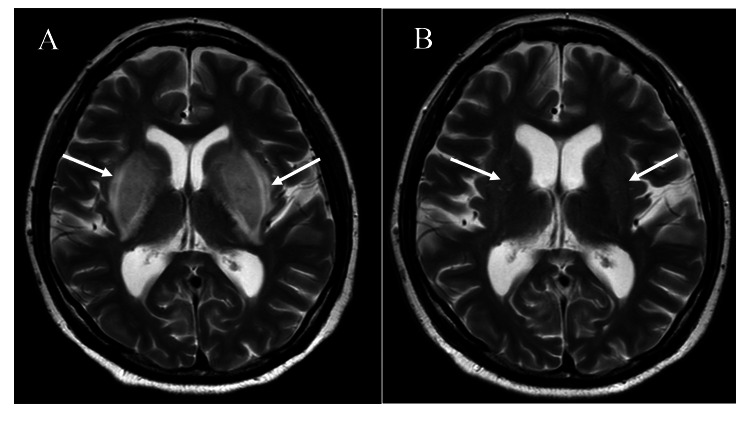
T2-weighted brain MRI performed in the emergency department (A) and follow-up brain MRI performed on hospital day 14 (B) A: Hyperintensity lesions were observed in the bilateral basal ganglia, presenting as the “Lentiform fork sign” B: The previously noted “Lentiform fork sign” had disappeared (arrows).
